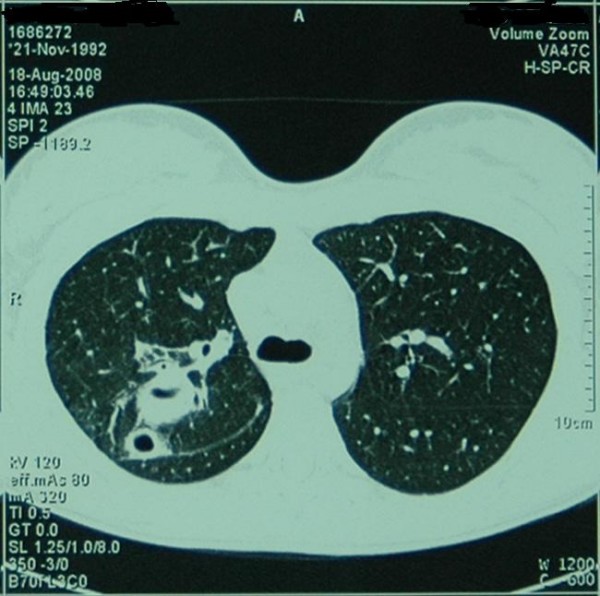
The computed tomography scan of this 16-y- old female revealed the presence of multiple abscesses at the right upper lobe.
